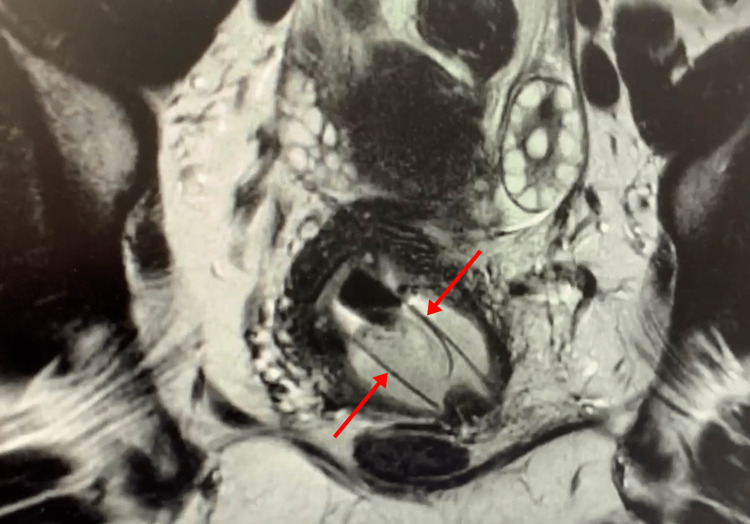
MRI displaying the anatomy of the cervix and upper vagina: a tubular foreign body is visualized within the endocervical canal and upper vagina. MRI, magnetic resonance imaging
